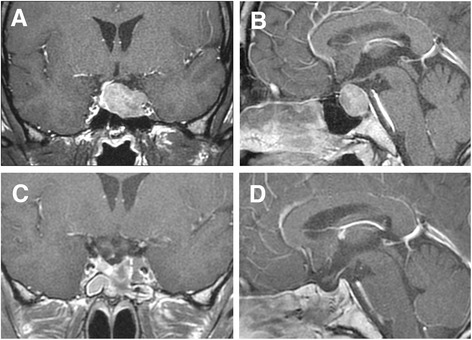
Magnetic resonance imaging (MRI) of the brain. Coronal (a) and sagittal (b) pre-operative gadolinium-enhanced T1-weighted MRI shows the presence of a macroadenoma. Coronal (c) and sagittal (d) post-operative MRI shows the presence of small residual tumors
